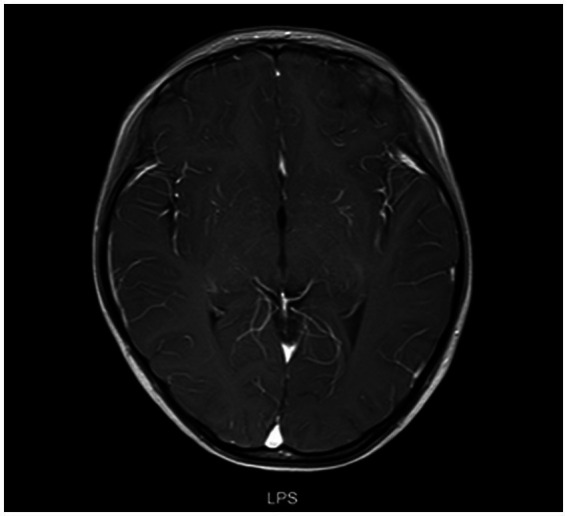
Cranial MRI results of a patient with meningitis who presented with fever. A female child, aged 78 months, was admitted to the hospital with fever for 9 days. At 19 days after admission, cranial enhancement MRI showed that the meninges of the right temporal pole were enhanced and no abnormality was found in the brain parenchyma.
